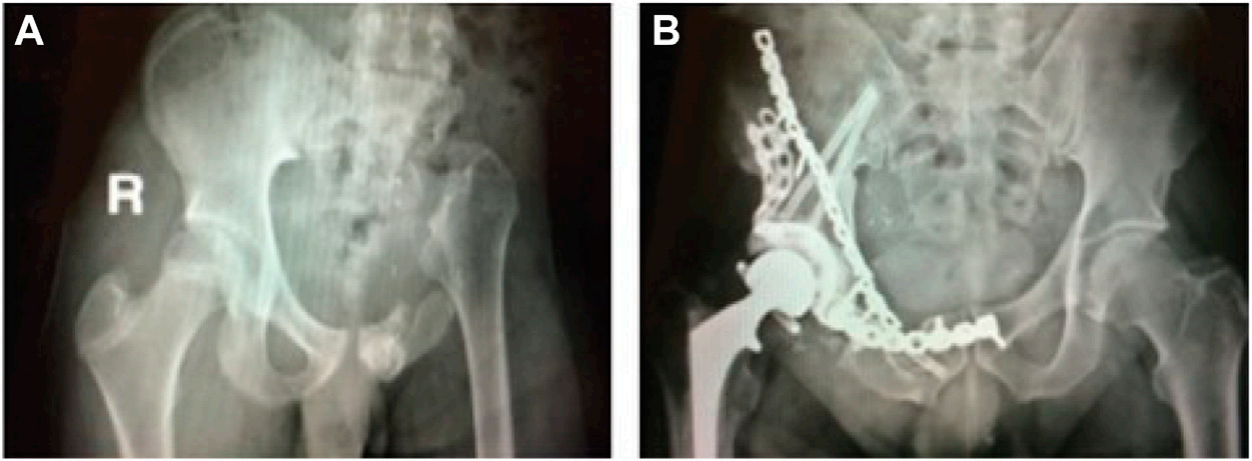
(A) Sample x-ray image of a pelvic cancer patient who received internal hemipelvectomy surgery with no reconstruction. (B) Sample x-ray image of a pelvic cancer patient who received internal hemipelvectomy surgery with allograph reconstruction and a total hip replacement. Images courtesy of Dr. Valerae Lewis, MD Anderson Cancer Center patient education DVD.
